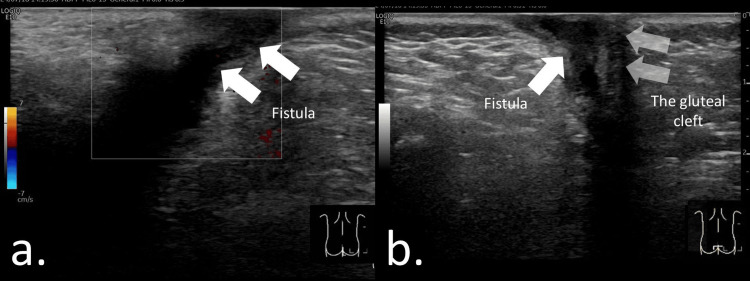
Fistula-in-ano at the 1 o’clock position in a nine-month-old boy. (a) A sagittal view of the anal canal was obtained by placing the probe parallel to the intergluteal cleft. A low-echoic linear structure consistent with a fistulous tract was visualized. No definite low-echoic fluid collection suggestive of an abscess was identified. Fistula-in-ano was diagnosed based on the sonographic findings. (b) A coronal view of the anal canal was obtained with the probe placed perpendicular to the gluteal cleft, demonstrating a low-echoic fistulous tract on the right side of the anal canal at the 1 o’clock position.
